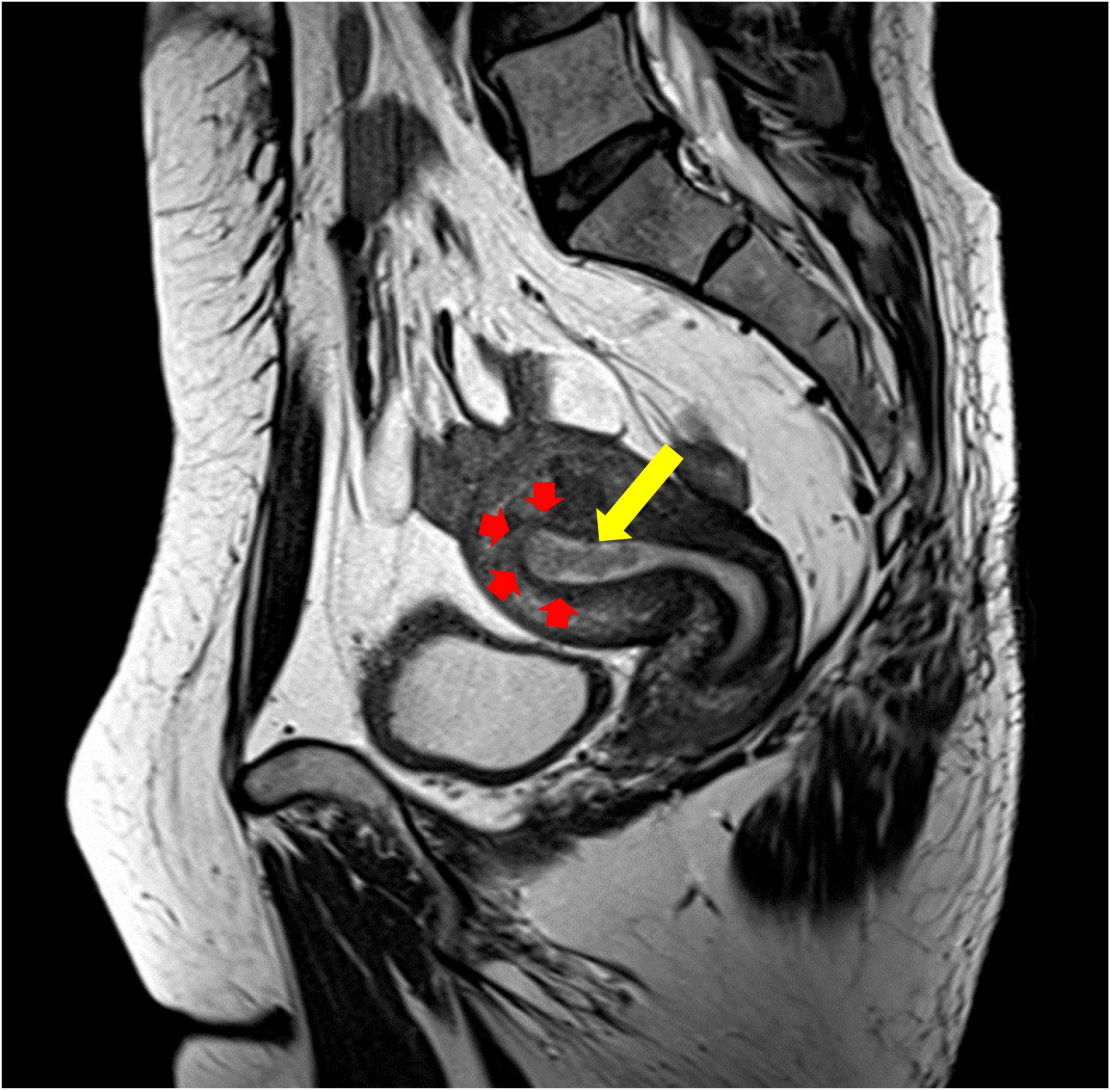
Pelvic magnetic resonance imaging (T2-weighted image). MRI revealed the presence of an endometrial tumor (yellow arrow) without myometrial invasion (red arrows).
